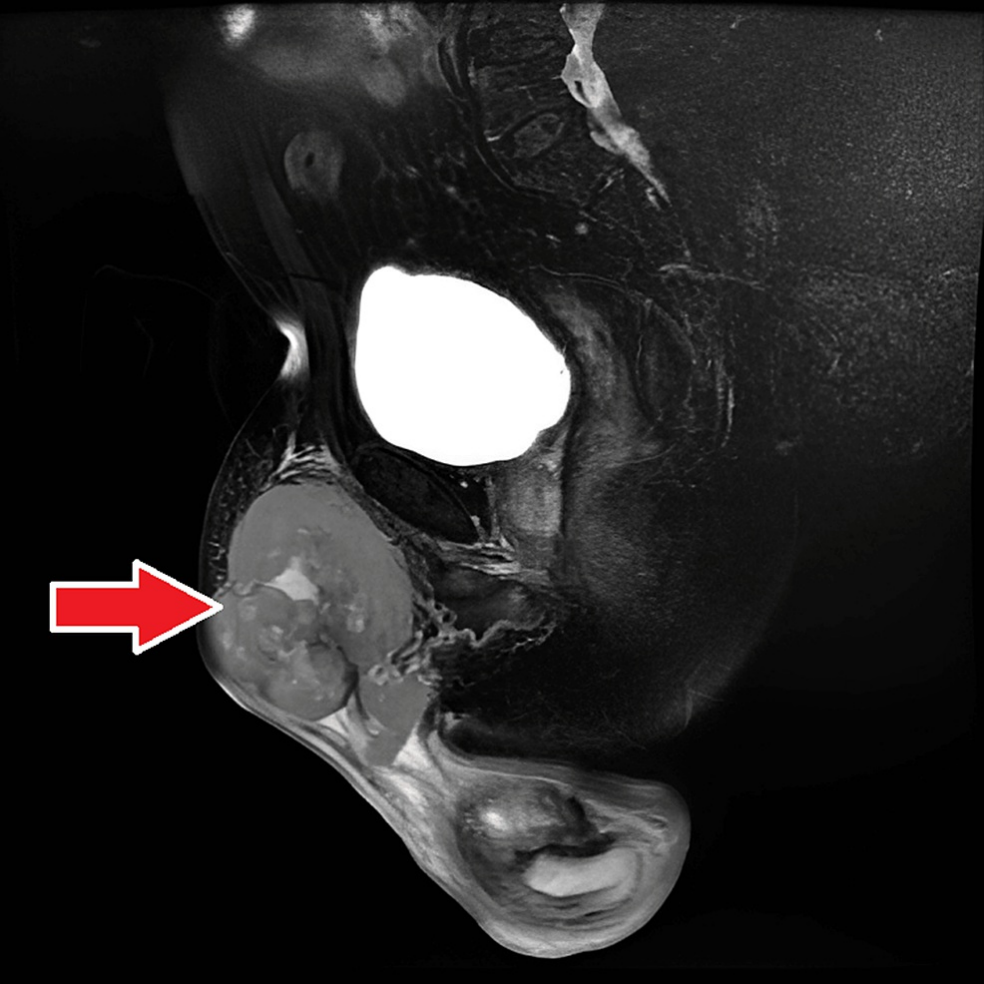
MRI - sagittal T2 FRFSE The image demonstrates a sagittal T2 FRFSE sequence isointense heterogenous mass lesion in the right inguinal region; the mass lesion is interspersed by a few hyperintense areas MRI: magnetic resonance imaging; FRFSE: fast recovery fast spin-echo
